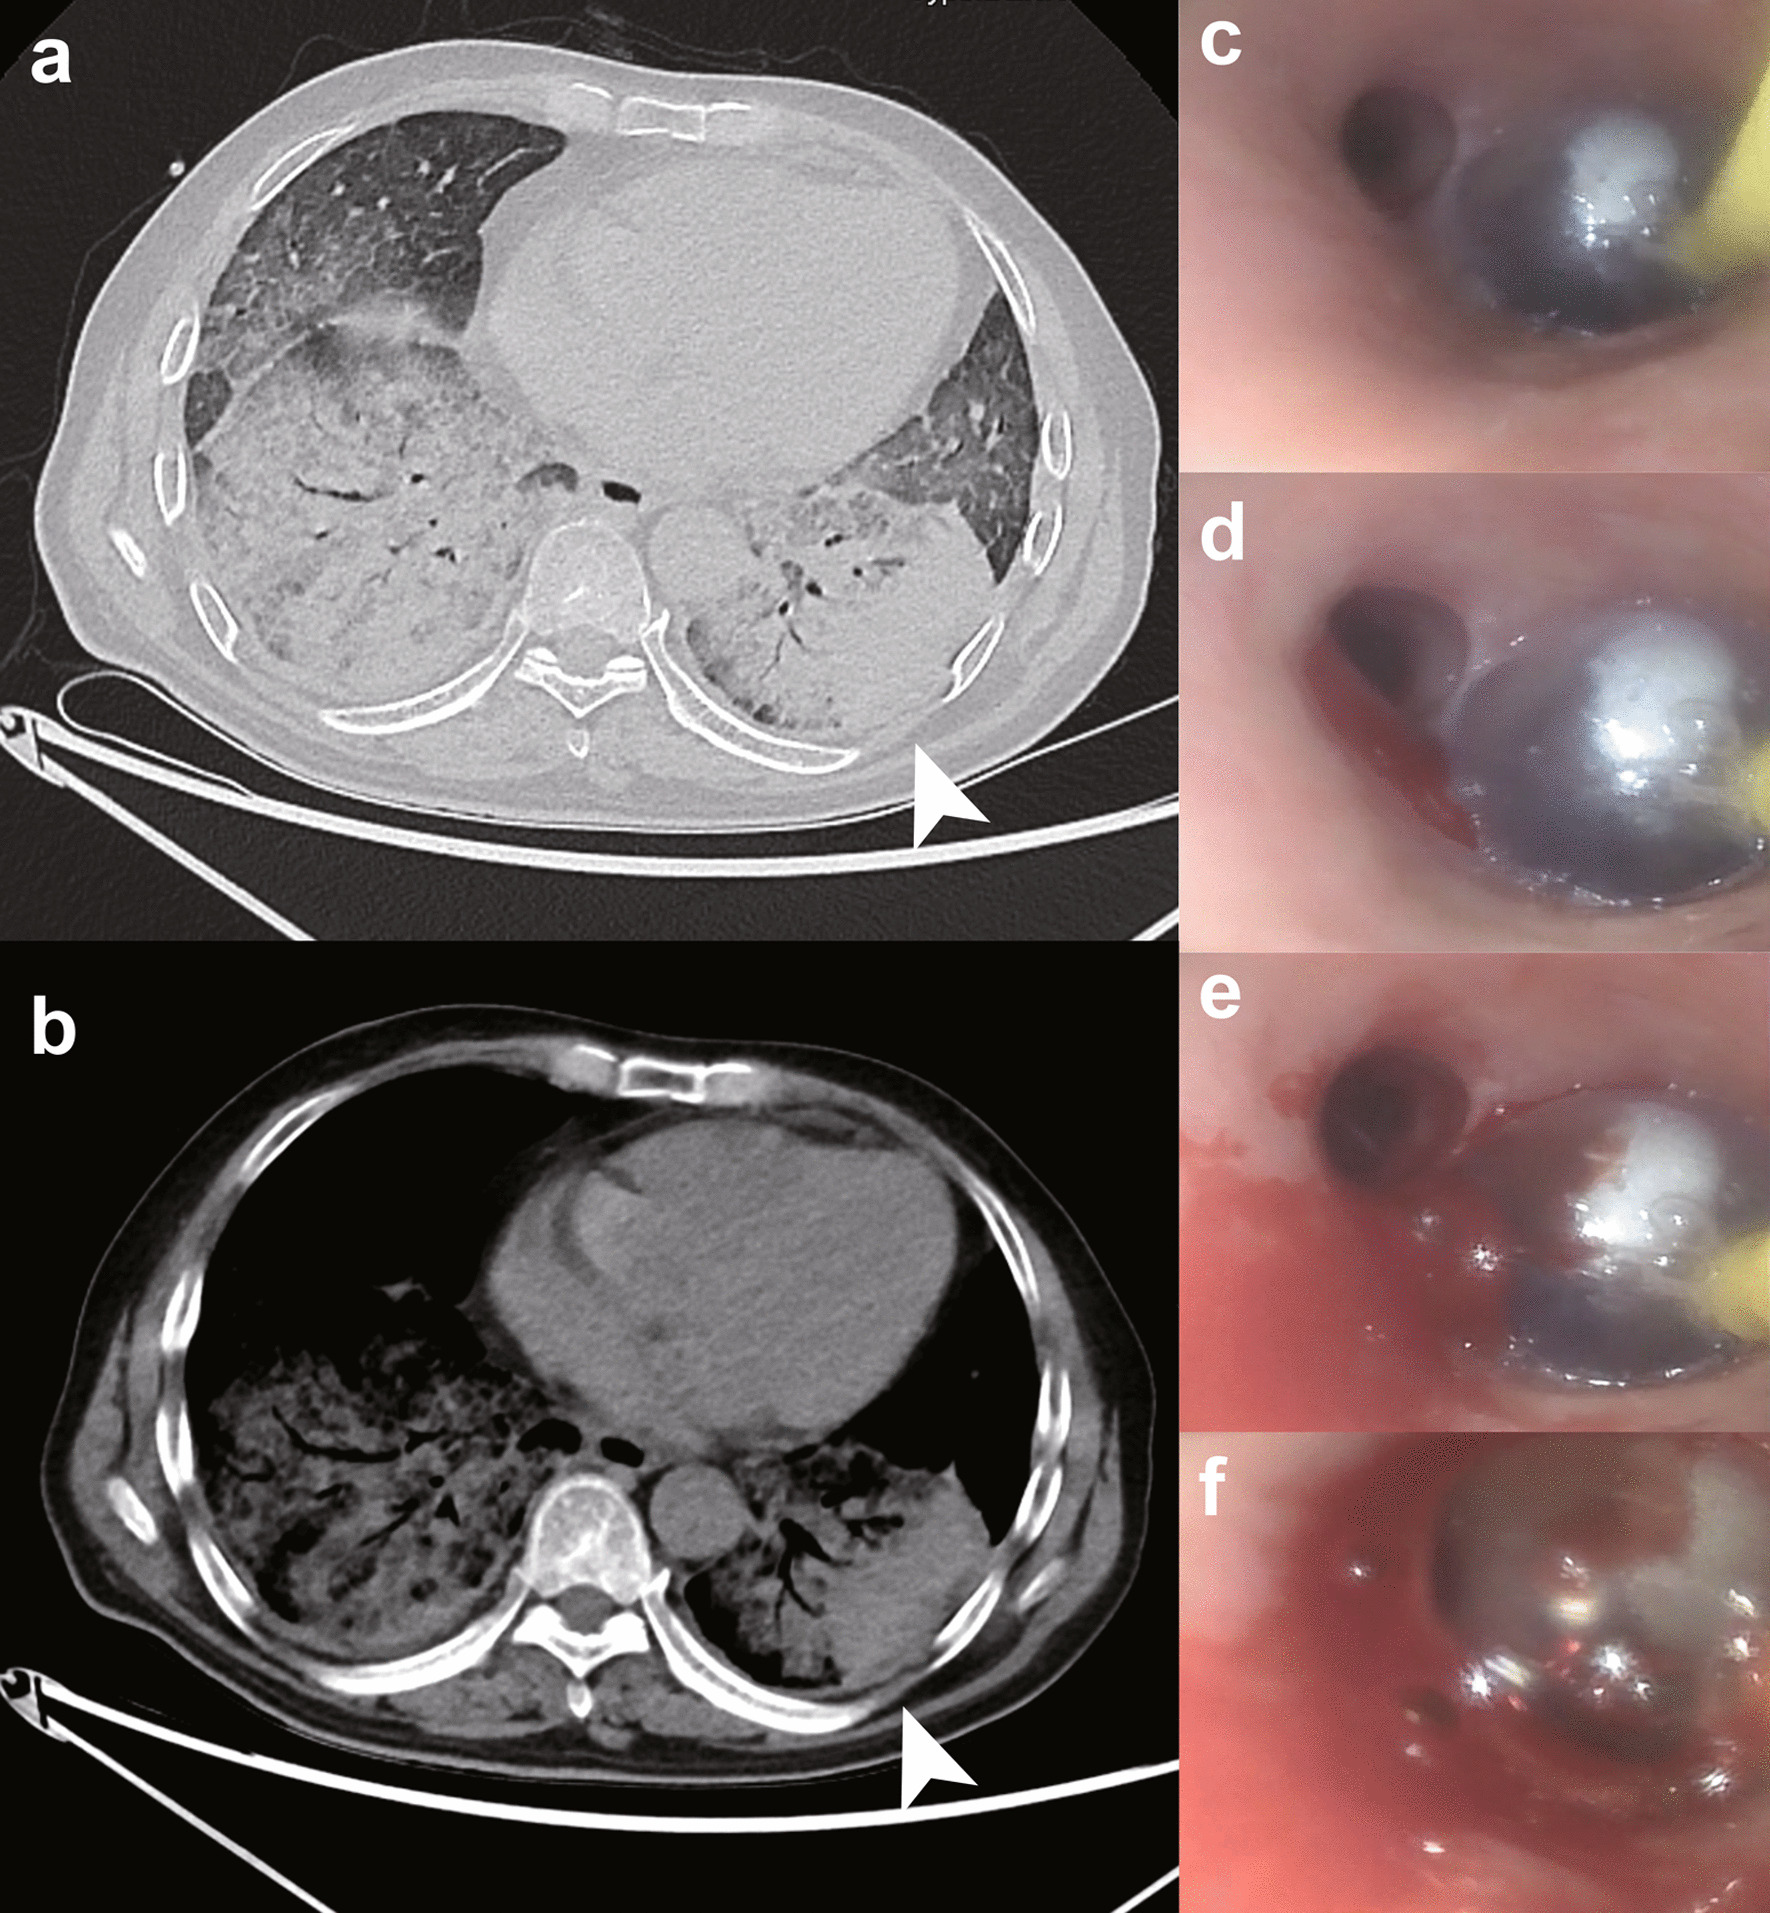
Biopsy site and vision of bronchial blocking under bronchoscopy for patient 1. a, b Chest CT of patient 1 showed diffuse patchy ground glass opacity with local interstitial changes over bilateral lung fields. Arrowheads indicate the site of puncture. c Bronchoscopy showing the endobronchial blocker was initially placed in the common basal segmental bronchus. d, e Then bleeding occurred in the dorsal segmental bronchus. f The endobronchial blocker was withdrawn backwards to inferior lobar bronchus to stop bleeding
